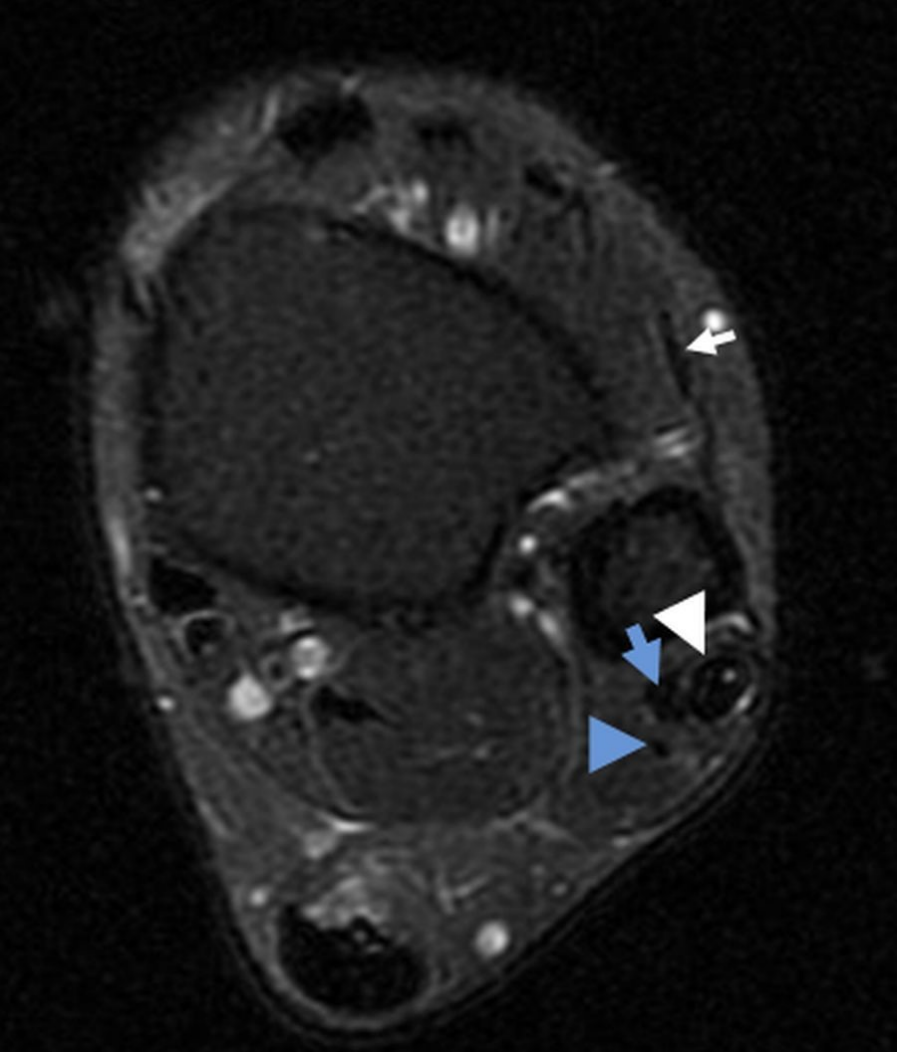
Peroneus Tertius and Quartus Axial T2-weighted image superior to the lateral malleolus demonstrates a normal peroneus tertius tendon (white arrow) anterior to the fibula. The peroneus longus tendon (white arrowhead) contains small foci of internal hyperintense signal, whereas the peroneus brevis (blue arrow) and quartus (blue arrowhead) tendons are normal in signal intensity.
